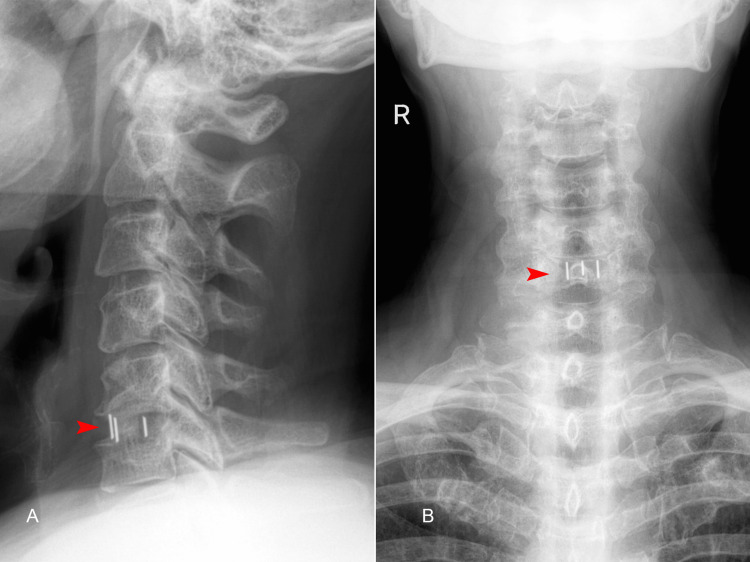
Postoperative X-ray of the cervical spine (A) lateral and (B) anteroposterior views, highlighting the interbody cage at the C5/C6 level (red arrows).
